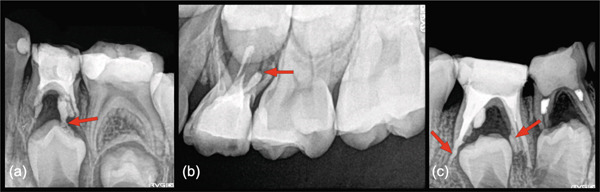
Examples of MTA extrusion directly after obturation. (a) (Case 7) Periapical radiograph of mandibular left first primary molar (No. L) after initial treatment showing furcation lesion, extrusion of compacted MTA expressed from both canals due to resorptive defects. Note the distal canal extruded MTA extending directly into the follicle and onto the occlusal surface of the permanent first premolar (arrow). (b) (Case 9) Radiograph of the left maxillary first primary molar (No. I) exhibiting voids in the mesio‐buccal and palatal roots. MTA extrusion and external inflammatory resorption are evident in the disto‐buccal root (arrow). (c) (Case 3) Periapical radiograph of the right and second mandibular primary molars (No. S,T). Both molars presented with previous pulpotomy treatments and demonstrated furcation pathosis. Postoperative image after MTA compaction No. T shows significant MTA overfill in the distal canal, extrusion into the distal furcation area and excess MTA in contact with the mesial aspect of the erupting second permanent premolar (arrows).
